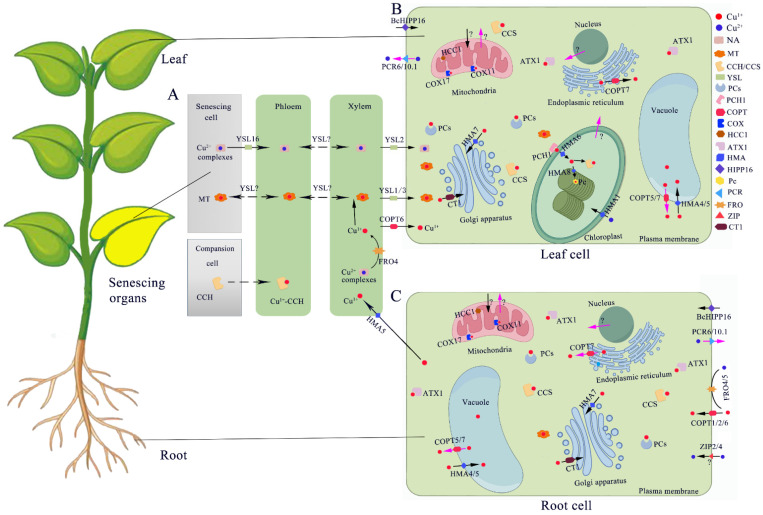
Overview of Cu transport mechanisms in plants. (A) Tissue specificity of Cu transporters, chaperones, and complexes. (B,C) subcellular localization of Cu transporters, chaperones, and complexes in root or leaf cells. In (B,C), black arrows indicate import into the cytosol, and purple arrows indicate export out of the cytosol. Proteins that undergo subcellular localization are only shown in yeast but not in plants and are indicated by a question mark. ZIP, Zn-regulated transporter; COPT, Copper transporter protein; FRO, Ferric reductase oxidase; HMA, Heavy metal ATPase; PCR, Plant cadmium resistance; PCH, Plas-tid chaperone; ATX1, Antioxidant protein; COX, Cytochrome coxidase; PCs, Phytochelatins; CCS/CCH, Cu chaperone; MTs, Metallothioneins; HCC1, the Arabidopsis homolog of the yeast mitochondrial copper chaperone SCO1; Pc, Plastocyanin; CT, MFS-type Cu trans-porter; YSL, Yellow stripe like; HIPP16, Heavy metal-associated isoprenylated plant protein 16.
